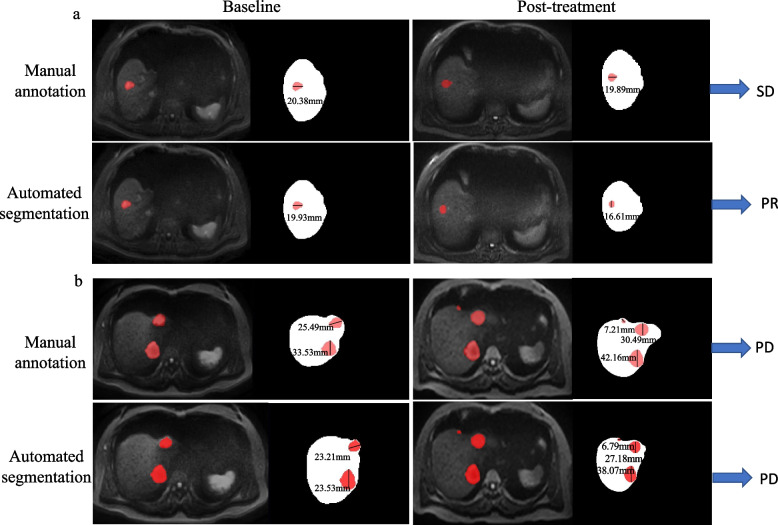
Example results of the treatment response assessment on DWI image. a liver metastasis from breast cancer in a 55-year -old female patient who was classified as having stable disease based on the manual liver metastasis segmentation but having partial response based on the automated liver metastasis segmentation; b liver metastases from rectal cancer in a 67-year-old male patient who was classified as showing progressive disease on the basis of manual and automated liver metastases segmentation
